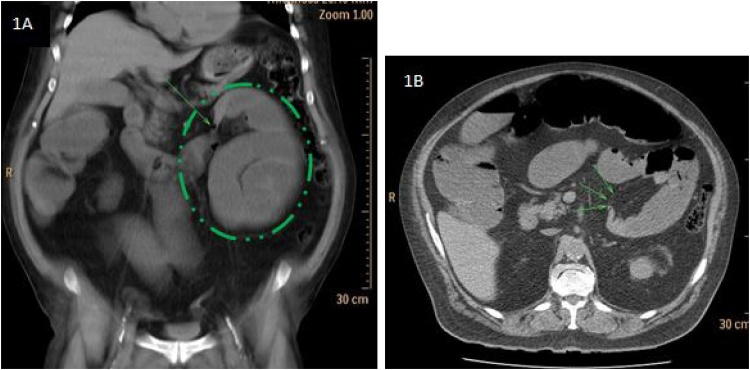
A and 1B: CT scan shows dilated small bowel loops with transition zone located left to the Treitz ligament (green arrow) and saclike appearance (green circle).
